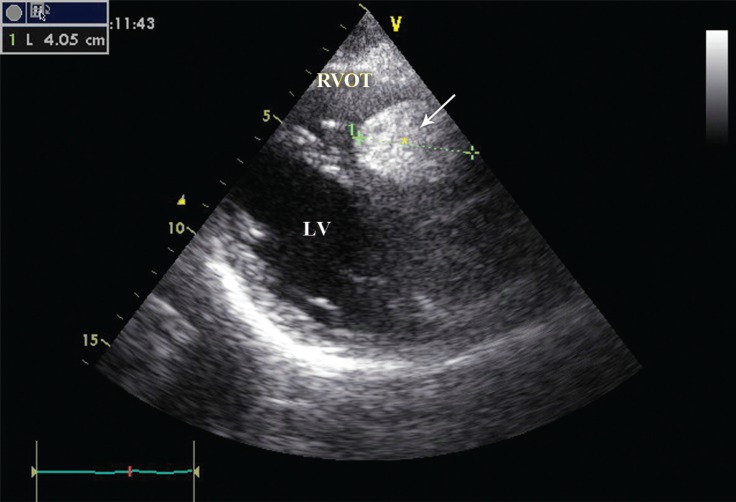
Two-dimensional parasternal long-axis view in transthoracic echocardiography shows a large mass in the right ventricular outflow tract (arrow).
